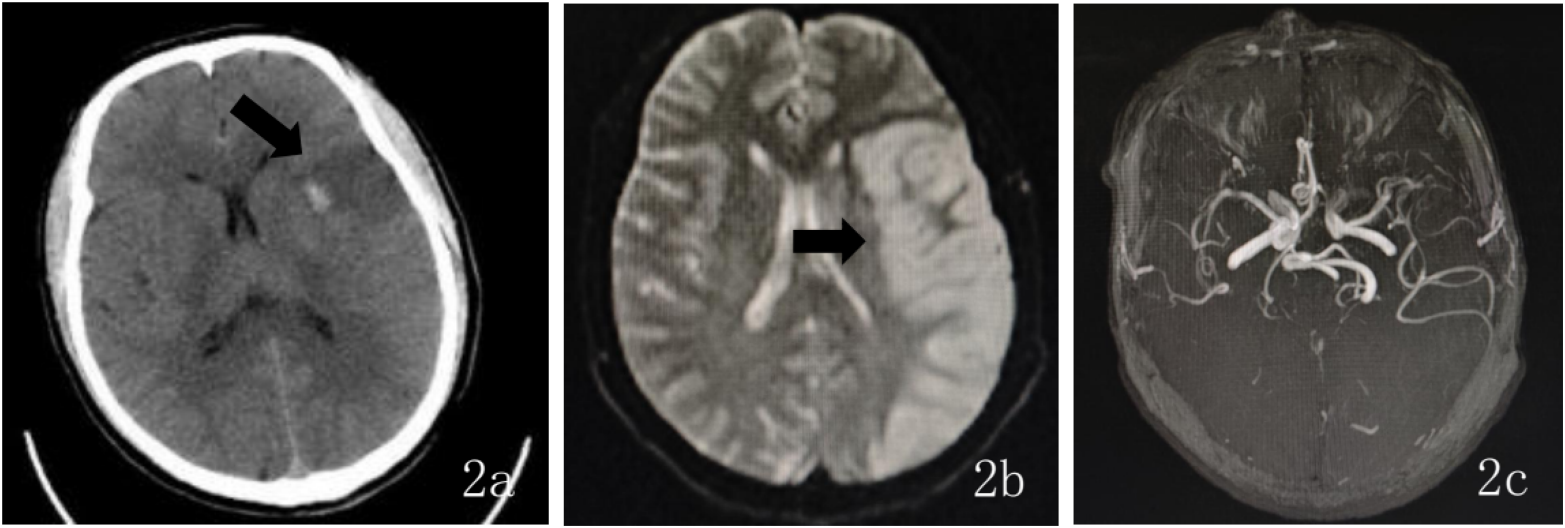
(a) Postoperative (October 17, 2024) head CT findings: axial CT shows a large area of slightly low-density lesions in the left frontal, temporal, and parietal lobes, and a small area of slightly high-density lesions in the left basal ganglia. (b) Postoperative (October 20, 2024) MR head imaging, including plain scan, diffusion-weighted imaging (DWI): Axial DWI shows high signal intensity in the left temporal, parietal, and insular lobes. (c) Postoperative (October 20, 2024) magnetic resonance angiography (MRA) finding: MRA shows no significant stenosis or occlusion of the intracranial major arteries.
